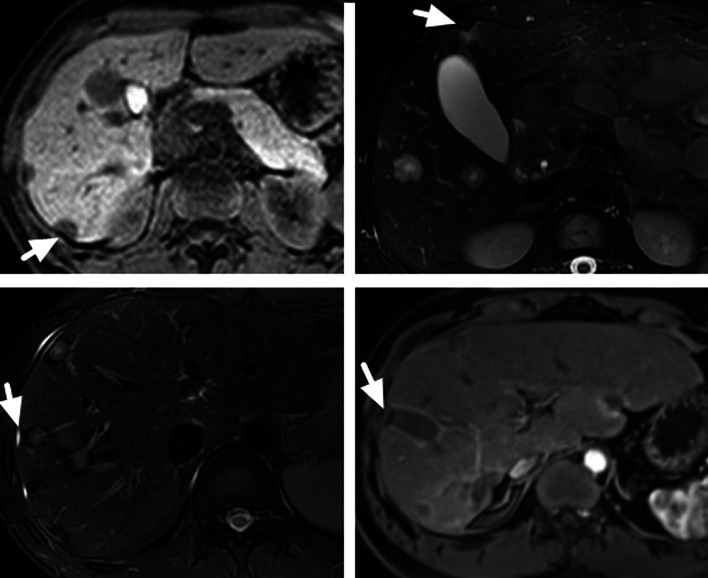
The appearance of capsular retraction on MRI images of 4 HEH patients (arrows)
